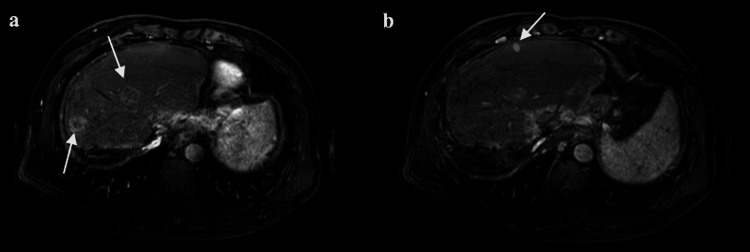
Arterial phase contrast-enhanced MRI revealed three hepatocellular carcinomas in the left lobe of the liver before proton beam therapy (a) Two high-intensity signal round-like nodules of 32 mm and 23 mm, respectively, in S4 and S2. (b) A 10-mm high-intensity signal round-like nodule in S2.
